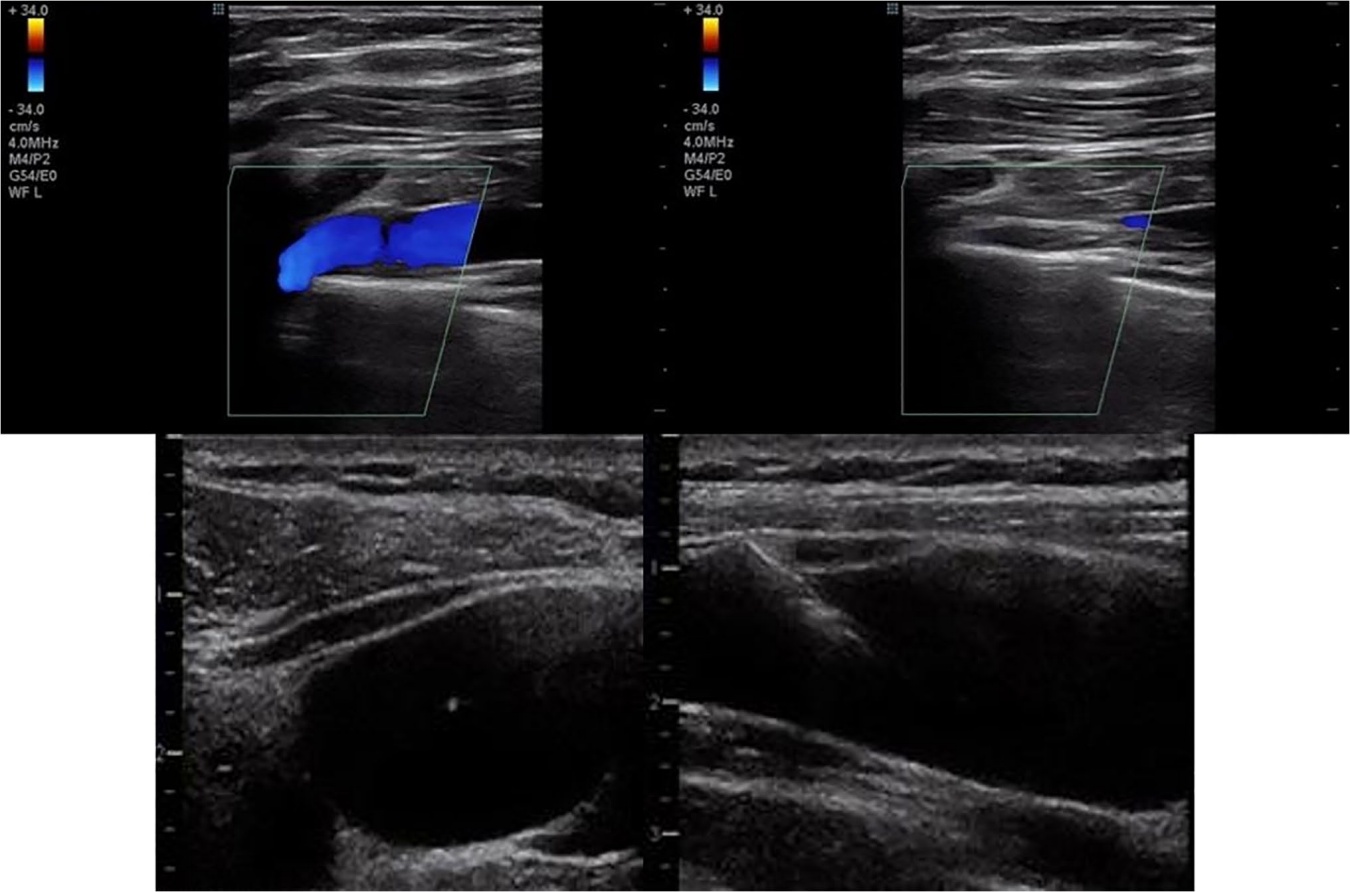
Upper left panel: transcutaneous images with color Doppler flow of left subclavian vein (blue vessel). Upper right panel: the same vessel was confirmed to be venous with ease of compressibility and digital pressure over the lumen. Lower panels (figure obtained with permission from common license) [41]. Transcutaneous ultrasound shows direct visualization of access needle insertion into a central venous vessel to a proper depth
